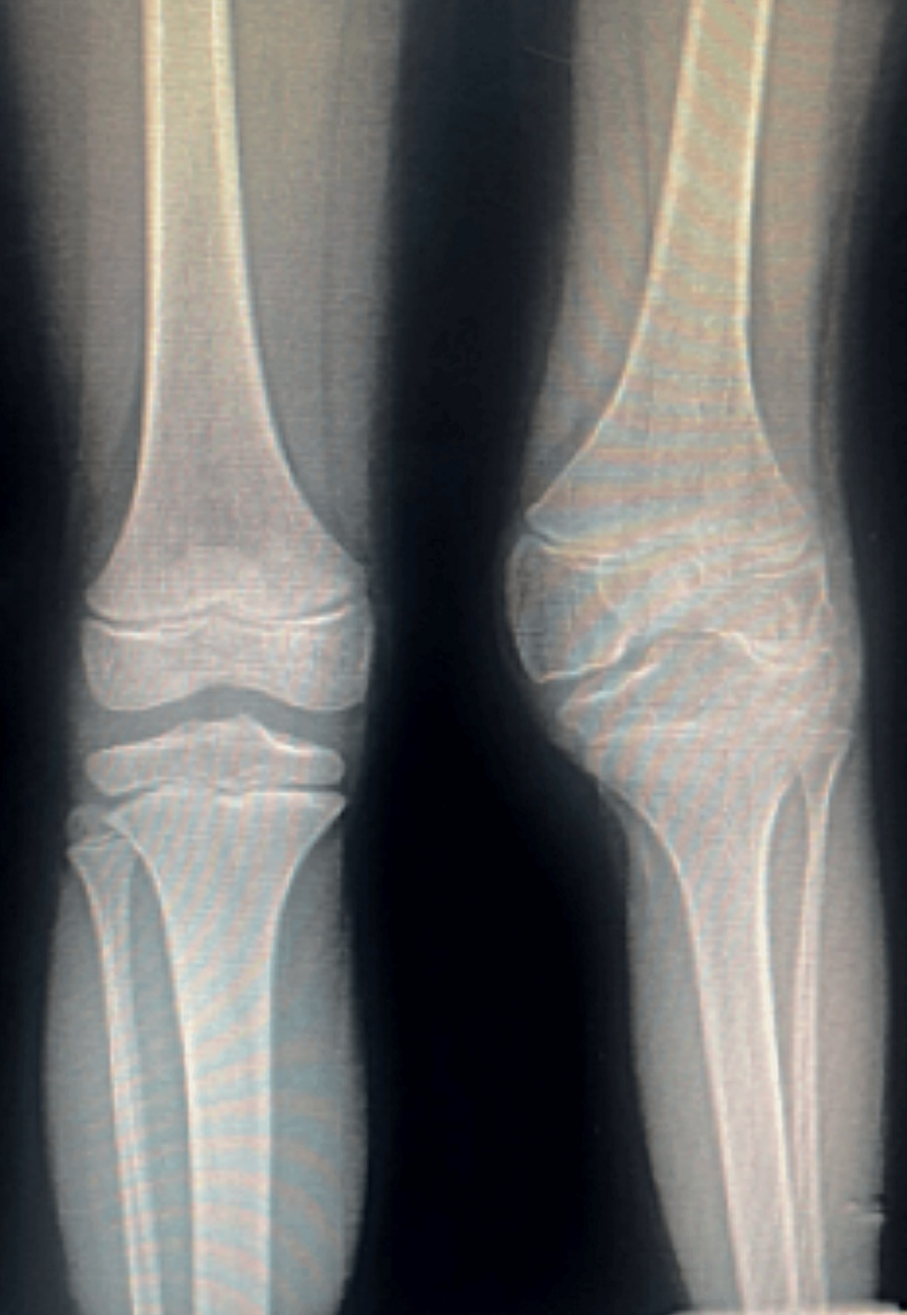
Preoperative anterior X-ray of the hemophilic knee
